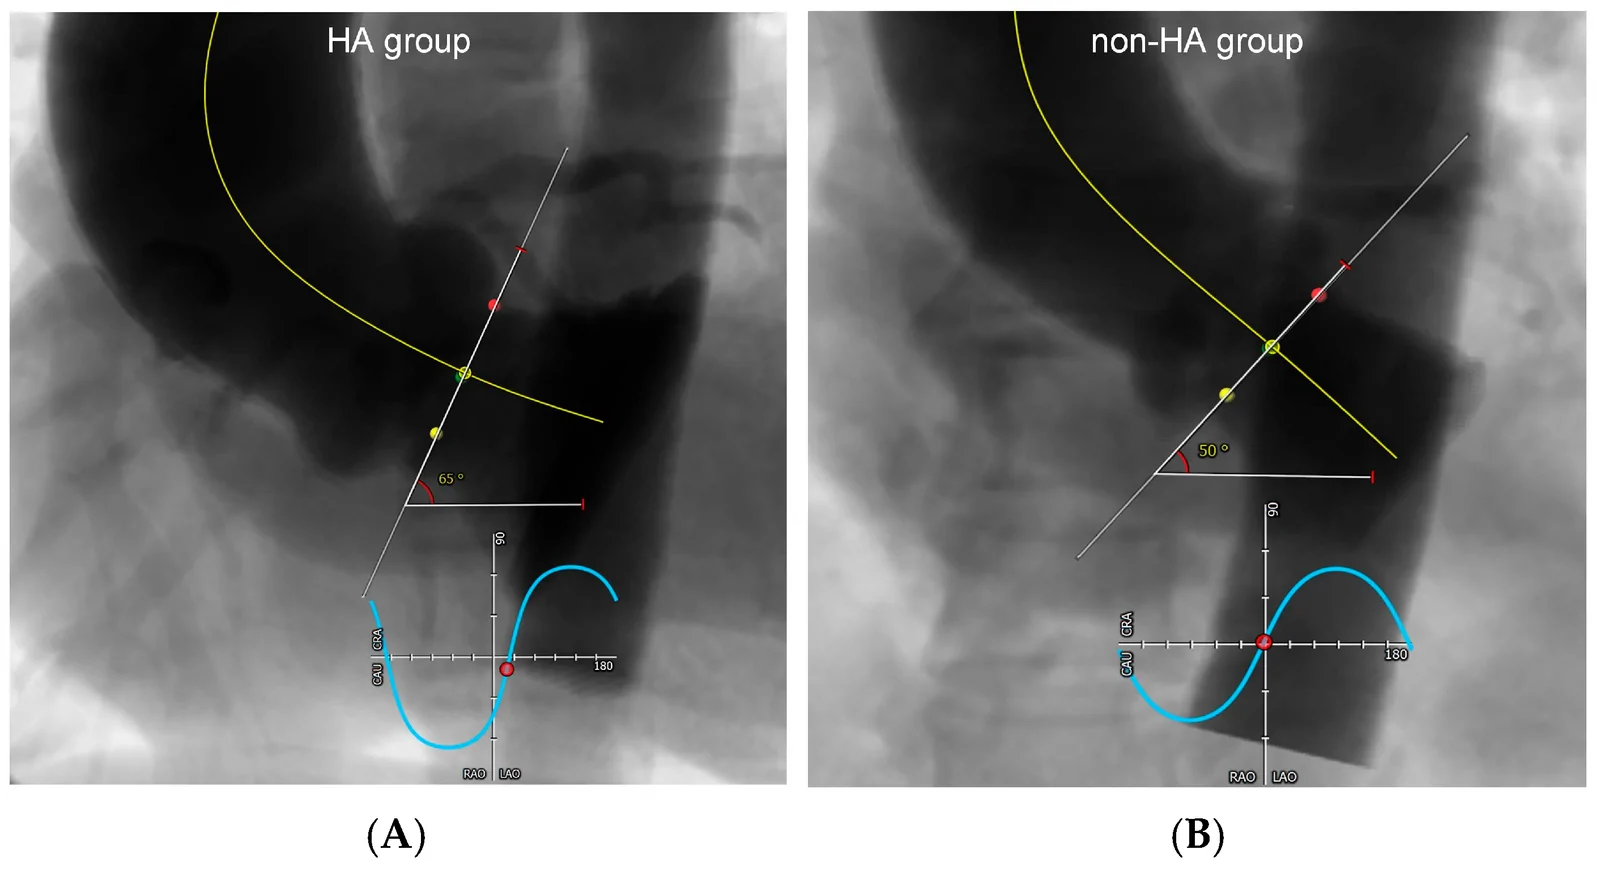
Measurement of the aortic angle on reconstructed multidetector computed tomography images. (A,B) Example of horizontal aorta and non-horizontal aorta. The oblique gray line represents the projection of the aortic annular plane, and the horizontal gray line represents the horizontal reference plane. The red arc and the corresponding numerical value indicate the measured aortic angle. The yellow curved line represents the reconstructed aortic centerline. The colored dots are software-defined reference points used for image reconstruction and angle measurement, whereas the blue curve and coordinate axes indicate the orientation of the reconstructed image. HA, horizontal aorta.
